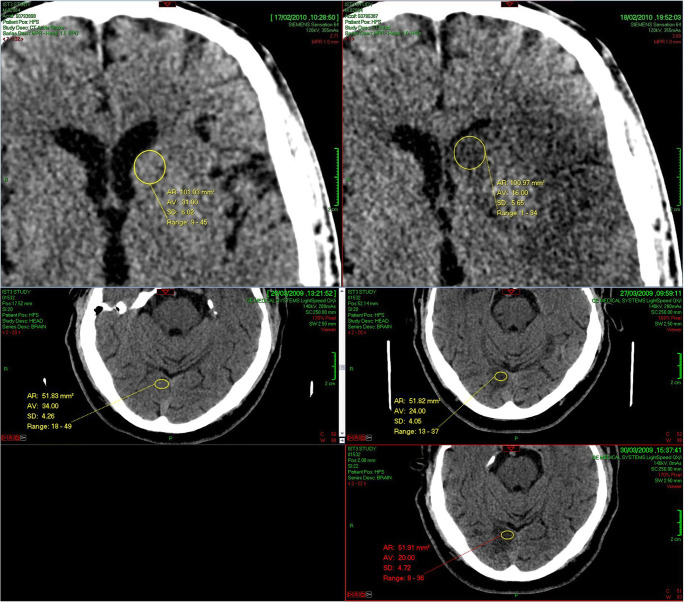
ROI placement within the same ischemic lesions on sequential thin-slice CT scans for two different patients. Note: Upper panel: Two sequential scan measurements from the same patient, left image at 175 min from stroke symptom onset, right image at 36 h from onset. Lower panels: Three sequential scan measurements from the same patient, left image at 120 min, middle right image at 22 h, and lower right image at 100 h from stroke onset
